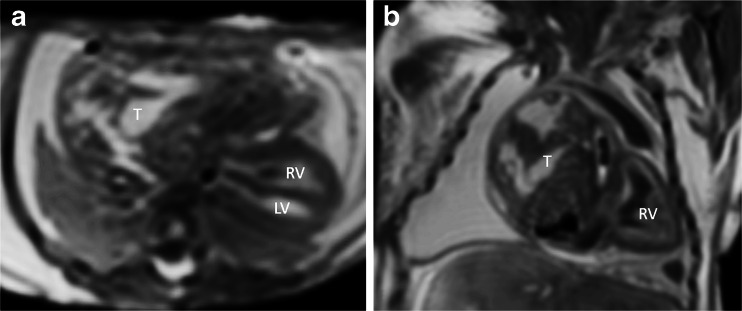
Fetal cardiac postmortem MRI in a 29-week gestation fetus shows a large cardiac teratoma (*T*) following unsuccessful in utero laser ablation. The large teratoma displaces the heart posteriorly and laterally on (a) axial and (b) oblique coronal T2-weighted images. *LV* left ventricle*, RV* right ventricle
